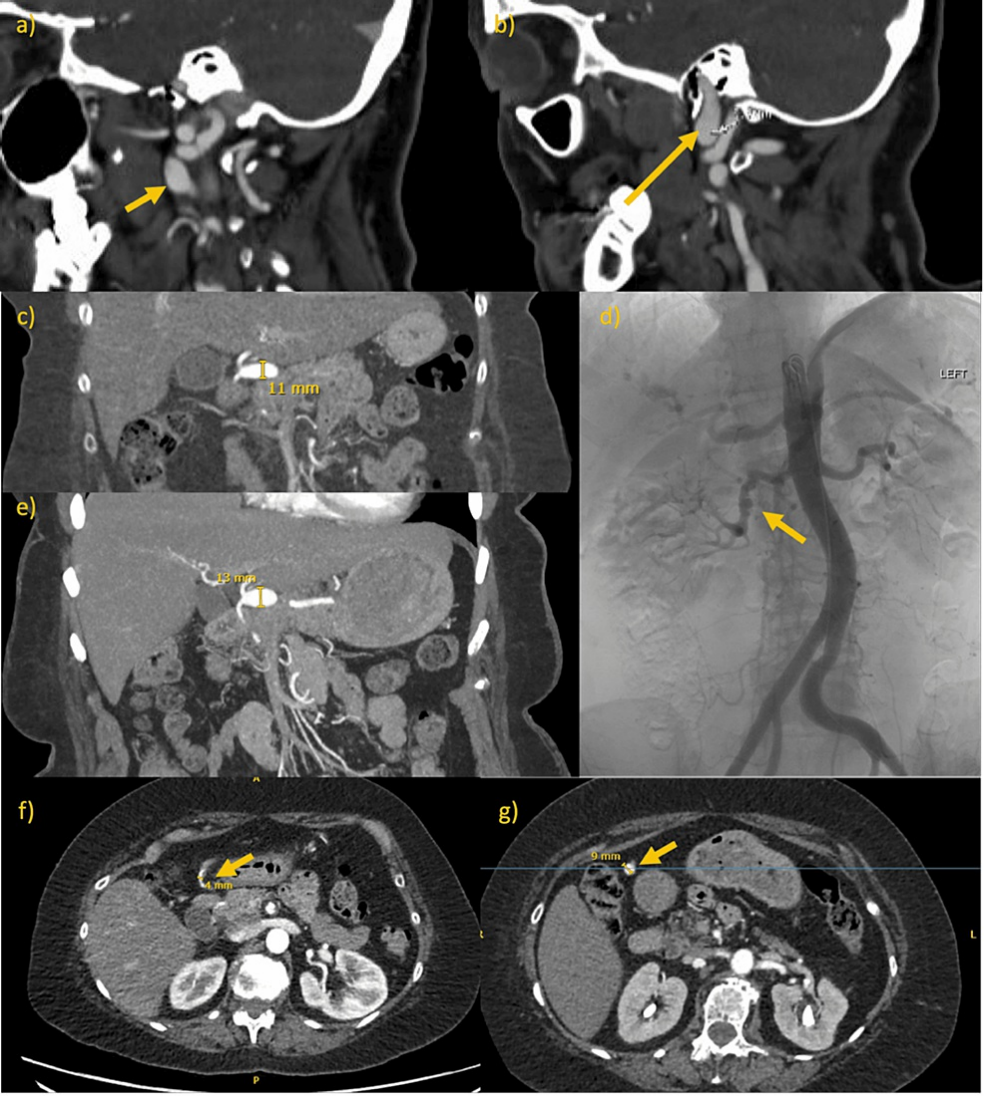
CTA (highlighted by arrows) demonstrating (a) right and (b) left significant distal cervical FMD and fusiform ICA aneurysm (yellow arrows). (c) CTA demonstrating 11mm CHA aneurysm in coronal plane. (d) Abdominal angiogram (highlighted by arrow) demonstrating evidence of FMD with beading of the right renal artery. (e) CTA performed nine months later demonstrating enlarged 13mm CHA aneurysm. CTA (highlighted by arrow) demonstrating GDA in axial plane on (f) presentation and at (g) nine months.
